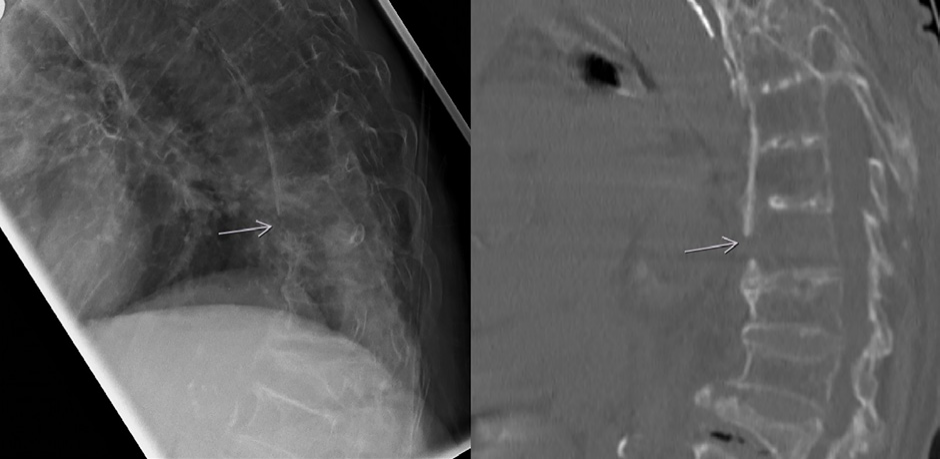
Fracture in an 88-year-old women presenting with persitent pain after a fall on the ground. Radiography (on the left) shows a disruption in the calcified ventral longitudinal ligament. This finding is confirmed on the CT-scan (on the right)
